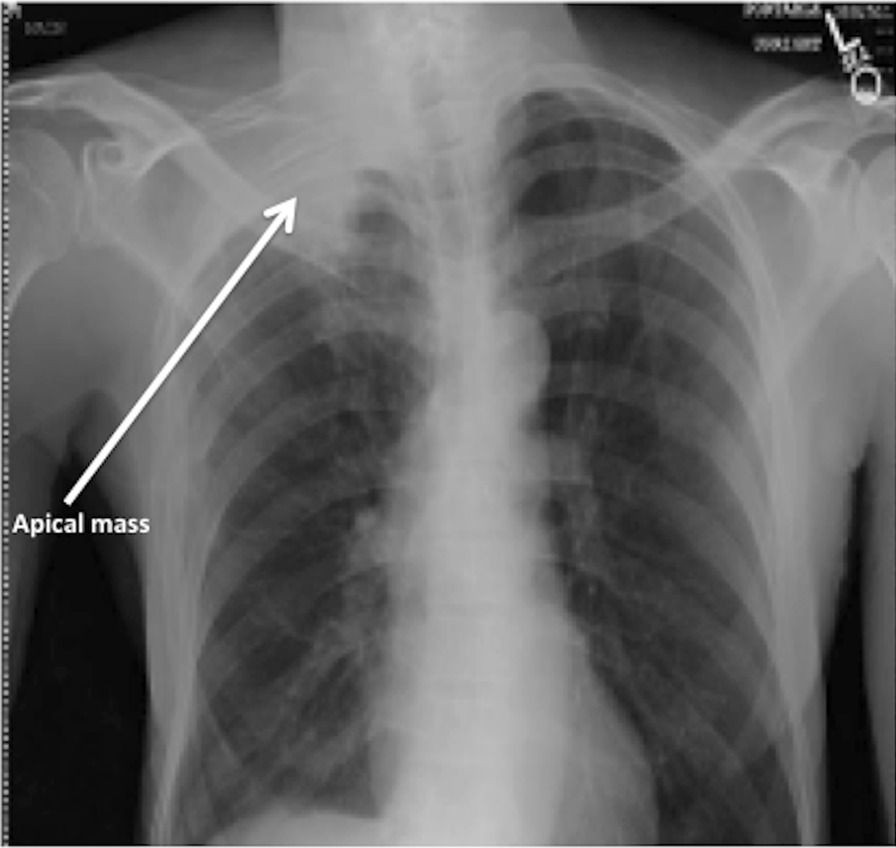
X-ray of the chest: an area of dense opacification in the right apical region; extensive bilateral upper lung bullous changes
